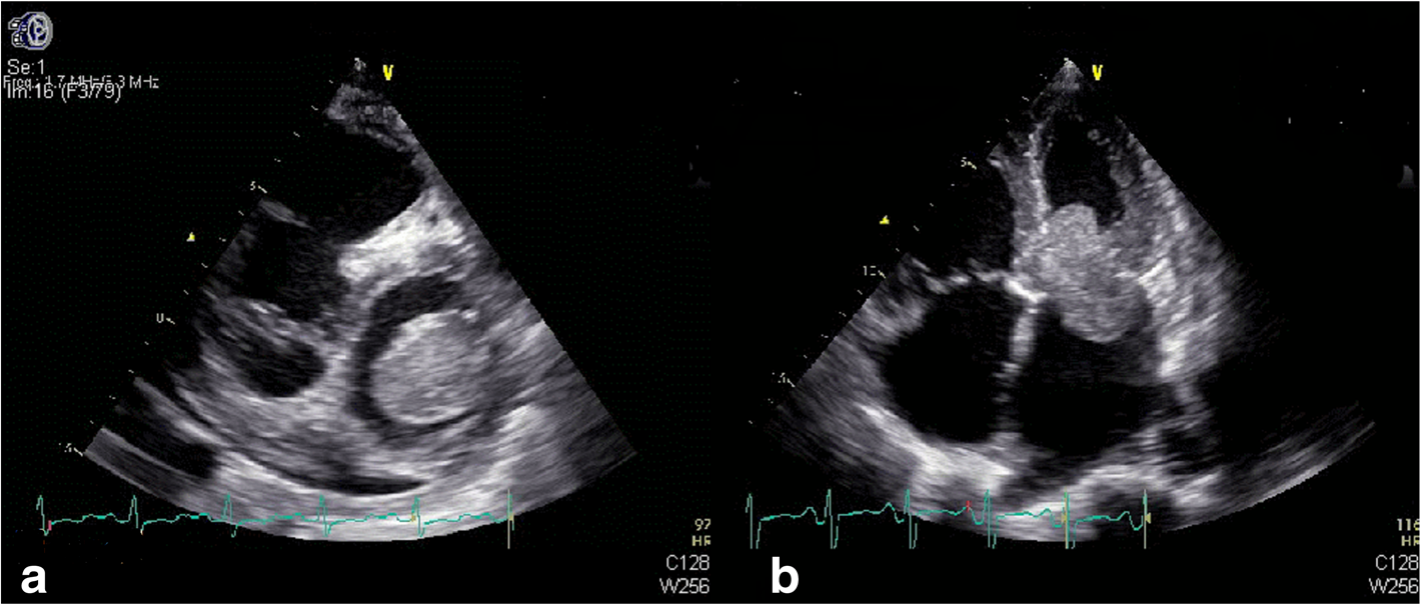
Preoperative echocardiography. a TTE showed a 50 × 35-mm solid tumour in the left atrium. b TTE showed that the tumour was adherent to the posterior annular region, obstructing the opening of the mitral valve
